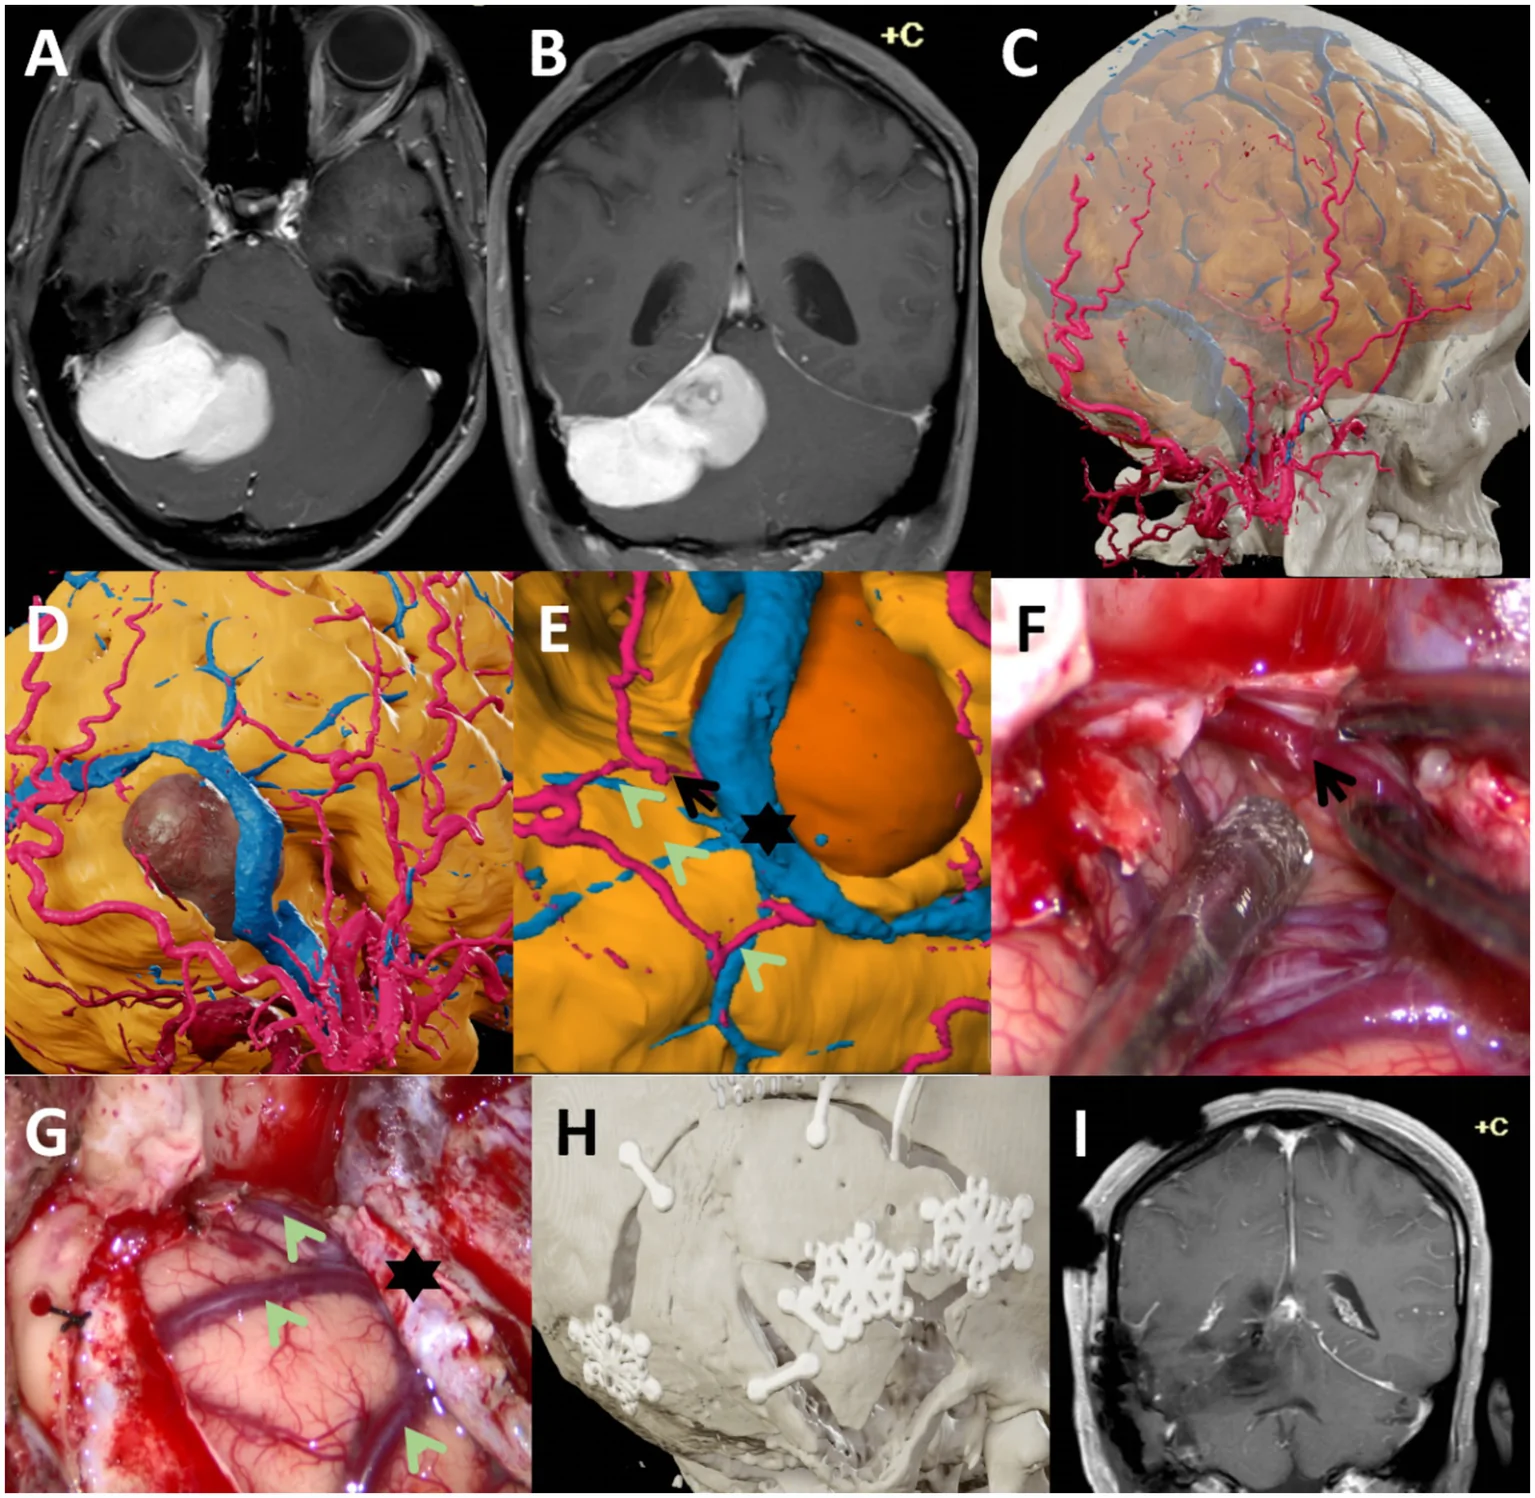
Tentorial-petrosal meningioma with complex arterial and venous relationships. (A,B) Preoperative MRI showing a right tentorial-petrosal meningioma. (C–G) MFI demonstrating arteries, veins, the sigmoid sinus, and the vein of Labbé. The vein of Labbé is indicated by the green arrow, the tumor-feeding artery from the middle meningeal artery by the black arrow, and the sigmoid sinus by the black asterisk. The preoperative reconstruction was visually consistent with intraoperative findings. (H) Postoperative cranial bone reconstruction. (I) Postoperative contrast-enhanced MRI showing Simpson grade I resection.
